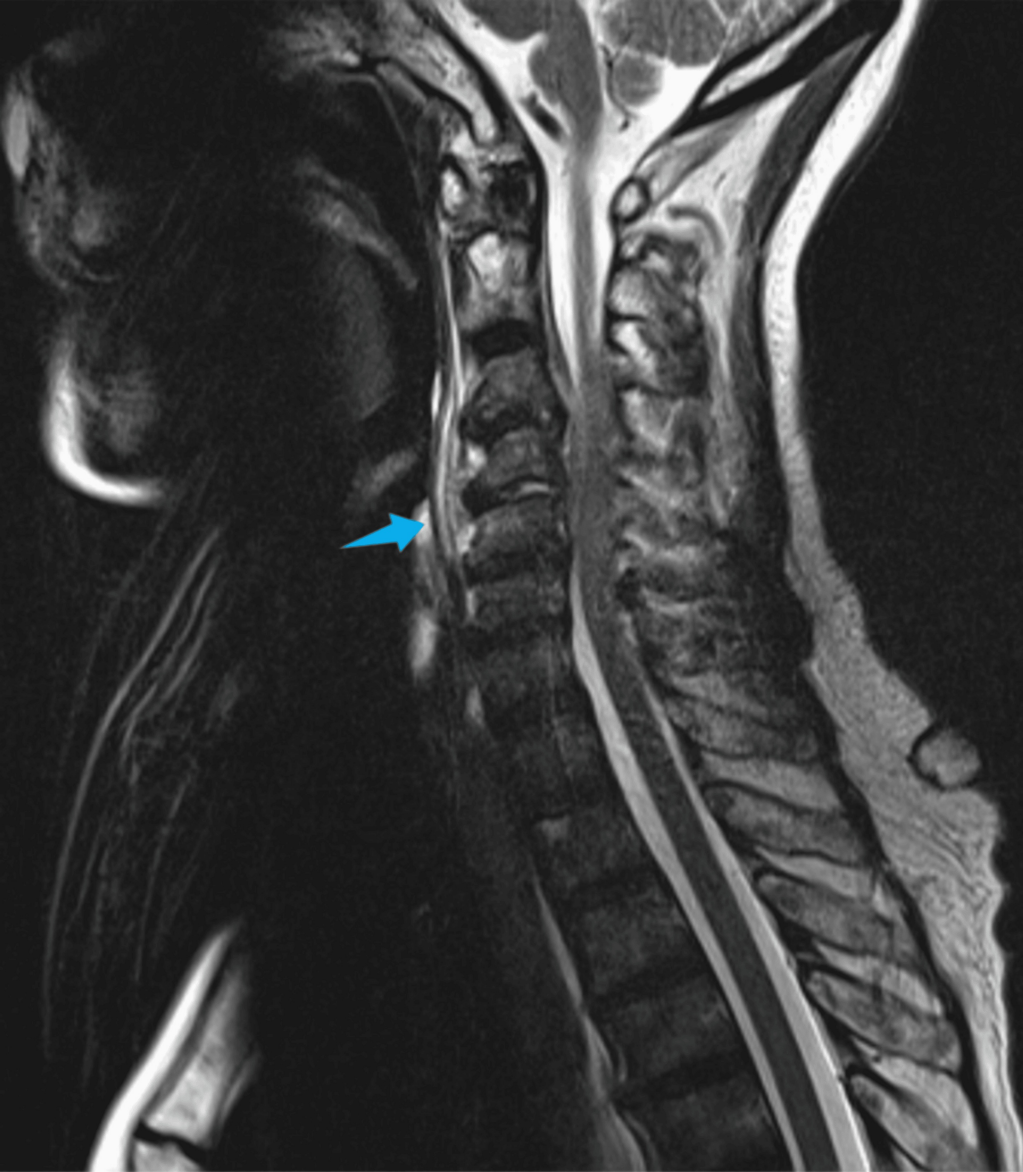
Sagittal T2-weighted MRI (June 2022) Inflammatory changes were identified in the C4-C5 intervertebral disc, as well as an anterior epidural collection with left paramedian predominance, between the body of C2 and the lower somatic platform of C5. This finding is consistent with the diagnosis of empyema (arrow). The collection extends through the foramina to the para and pre-vertebral space adjacent to the bodies of C3, C4, and C5.
